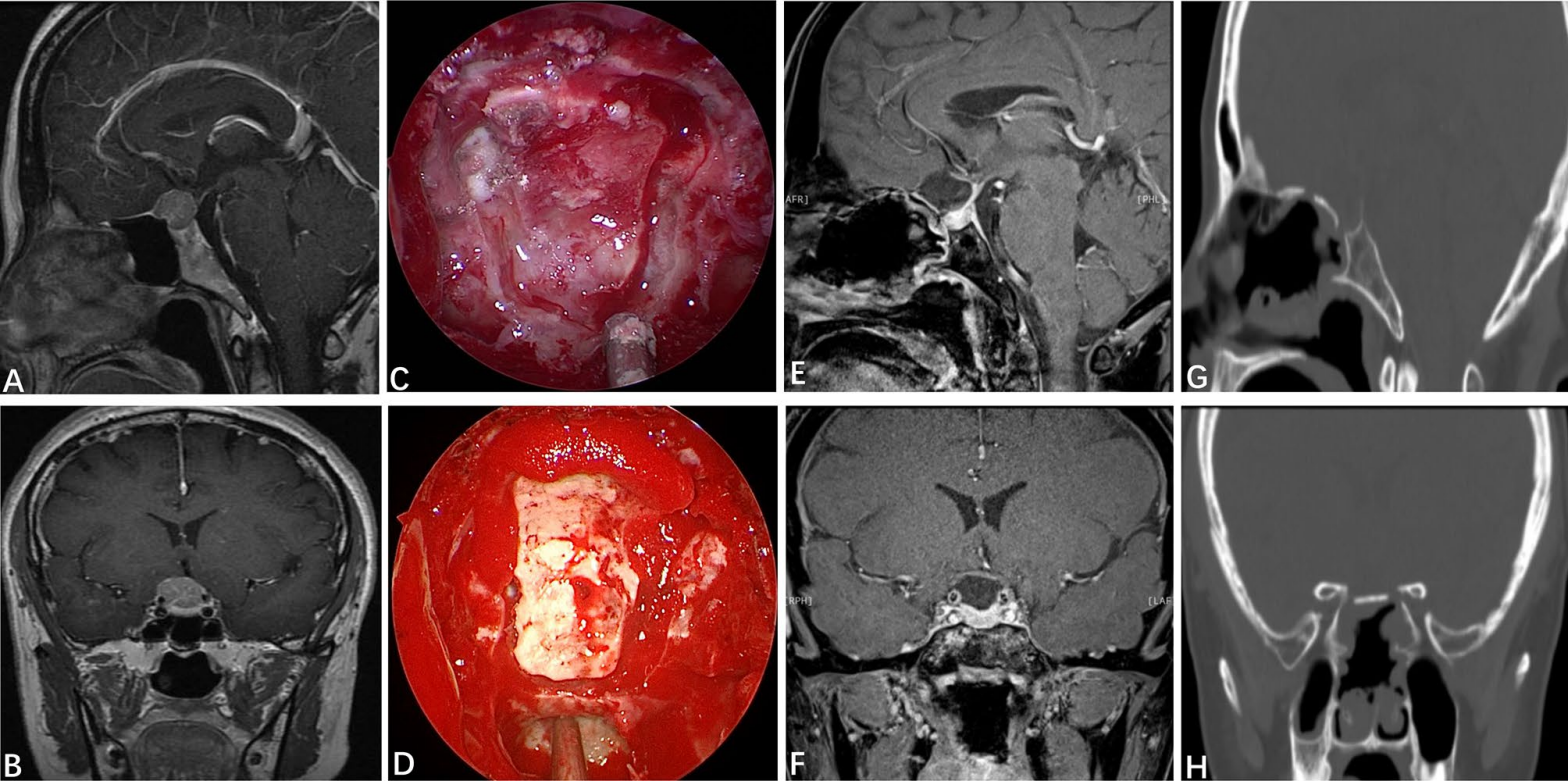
Reconstruction of the skull base with an autogenous in situ bone flap. (A,B) Preoperative contrast-enhanced MRI of the sellar region indicated that the tumor was to the right, and the structure of the optic nerve was unclear. (C) Formation of the autogenous in situ bone flap intraoperatively. (D) Autogenous in situ bone flap for skull-base reconstruction. (E,F) Three months after the EEEA, MRI of the sellar region indicated that the tumor had totally resected, and that the optic nerve, pituitary stalk and pituitary gland were intact. (G,H) Three months after surgery, CT of the skull base indicated that the bone flap was well restored, and the osseous anatomic structure of the skull base had been restored.
